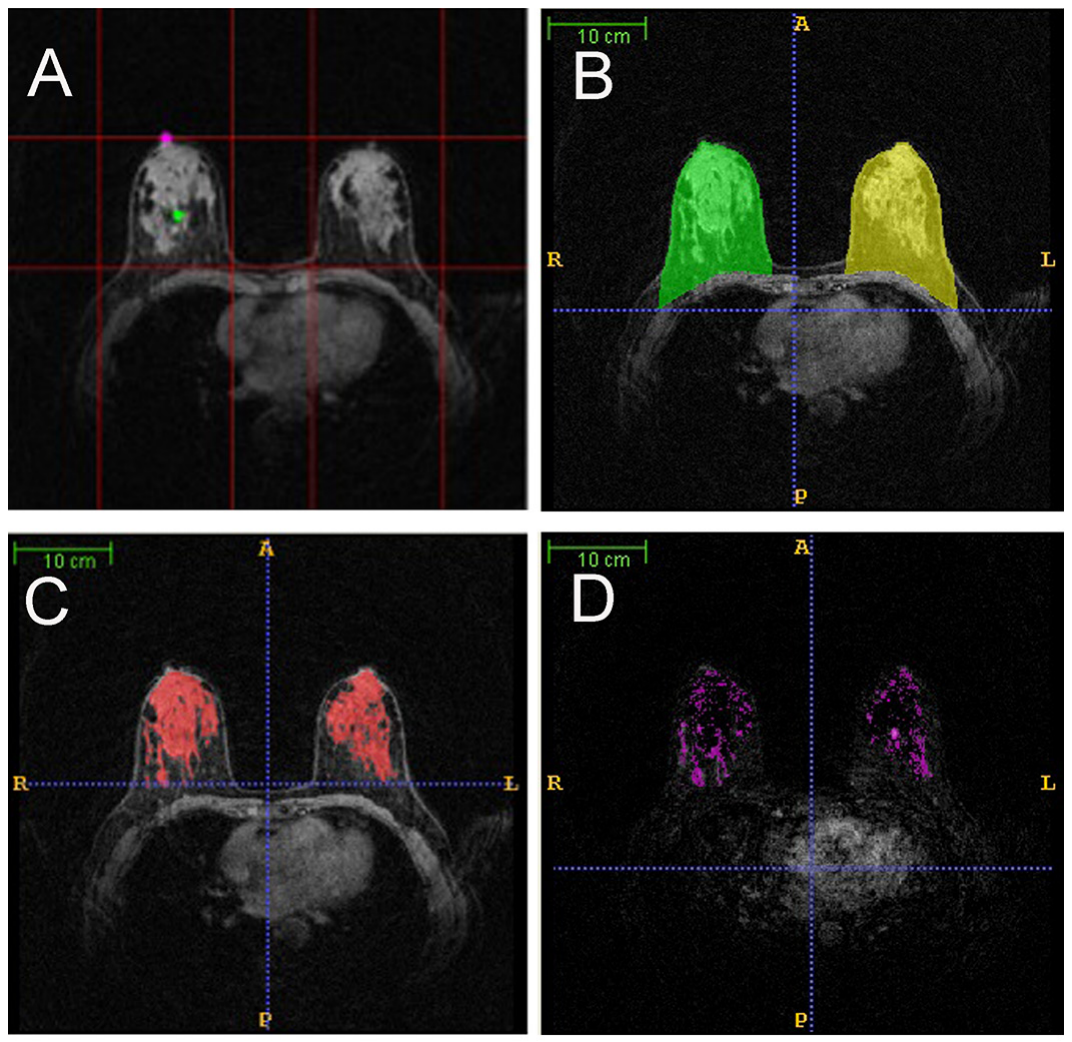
Magnetic resonance imaging analysis A. volume of interest (VOI) of breasts; B. whole breast segmentation (green for the right breast and yellow for the left breast); C. fibroglandular tissue segmentation (red); D. enhanced fibroglandular tissue segmentation (pink).
